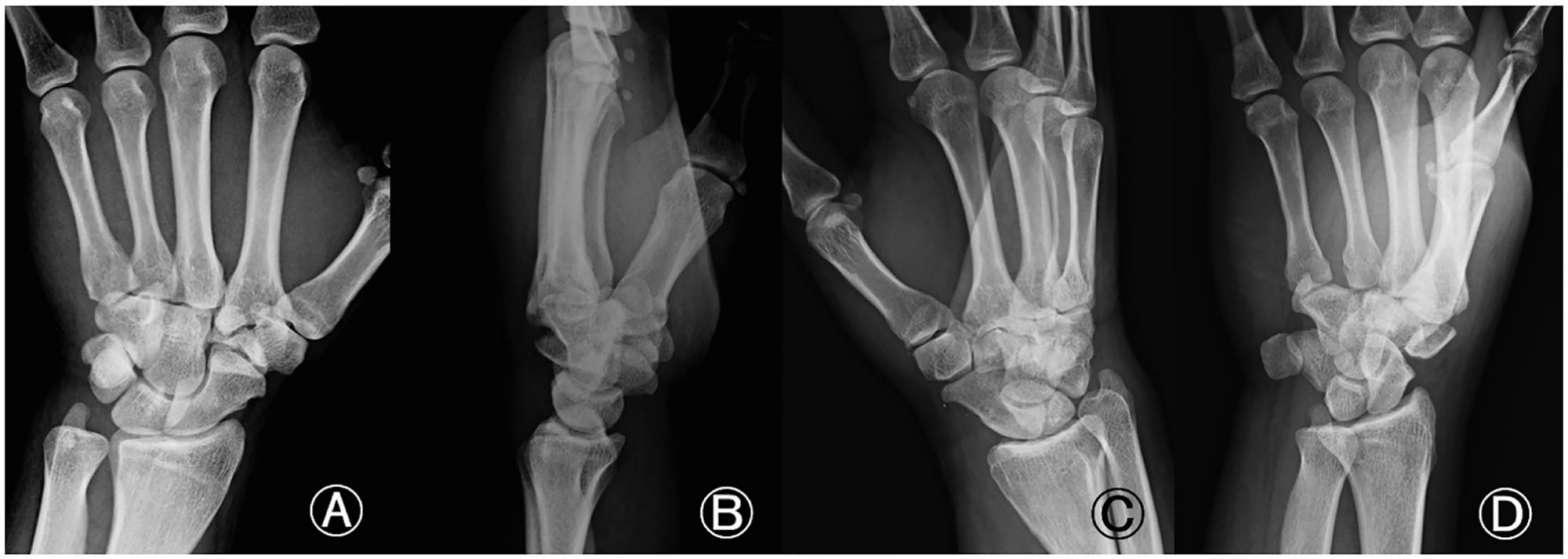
Isolated left trapezoid coronal shearing fracture in a 40-year-old male. (A) An X-ray of the wrist joint in the anterior-posterior view showing an irregular shape of the left trapezoid. (B) The lateral view in supination raised suspicion for a coronal shearing fracture of the trapezoid. (C) Oblique views recorded during pronation, showing no apparent findings. (D) The oblique view in supination clearly shows the fracture. No other carpal fractures are visible.
